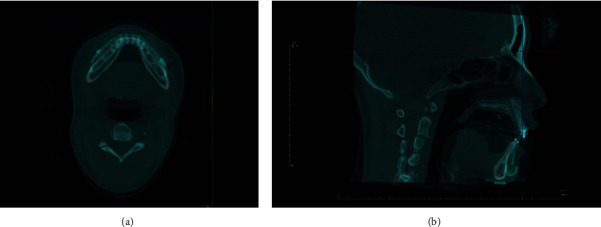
The superimposition of the PAS between *T*1 and *T*0 in the 3D CT image. (a) The superimposition in the axial view between *T*1 and *T*0. (b) The superimposition in the sagittal view between *T*1 and *T*0.
